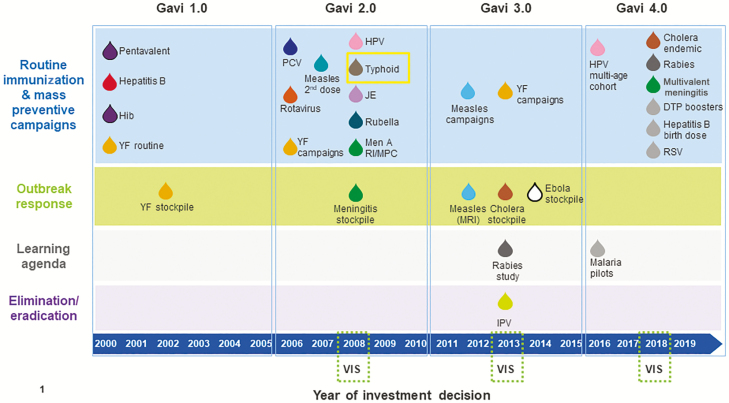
Timeline of historical Gavi investment decisions for new vaccine support, 2000–2018. Abbreviations: DTP, diphtheria, pertussis, and tetanus; Hib, *Haemophilus influenzae* type b; HPV, human papillomavirus; IPV, inactivated poliovirus vaccine; JE, japanese encephalitis; MRI, Measles & Rubella Initiative; PCV, pneumococcal conjugate vaccine; RI/MPC, routine immunisation/mass preventive campaigns; RSV, respiratory syncytial virus; VIS, Vaccine Investment Strategy; YF, yellow fever.
